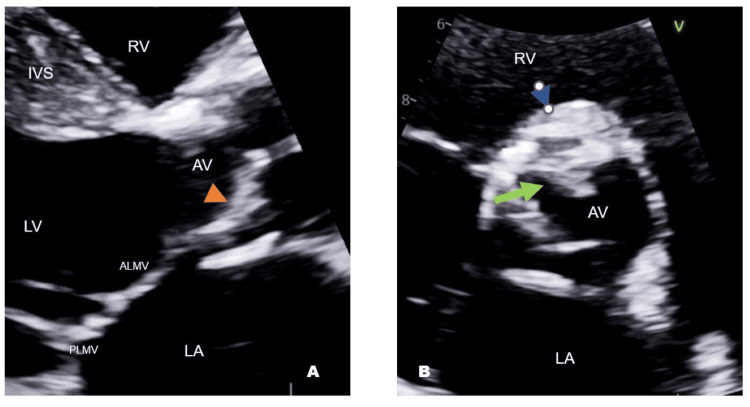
2D transthoracic echocardiogram, multiplane parasternal views of the bioprosthetic aortic valve. There is a thickening prosthesis with irregularly shaped, echo-dense masses with independent oscillatory motion more evidently attached to the right coronary cusp (arrows). ALMV: anterolateral mitral valve leaflet; PLMV: posterolateral mitral valve leaflet; LA: left atrium; RV: right ventricle; IVS: interventricular septum; LV: left ventricle
